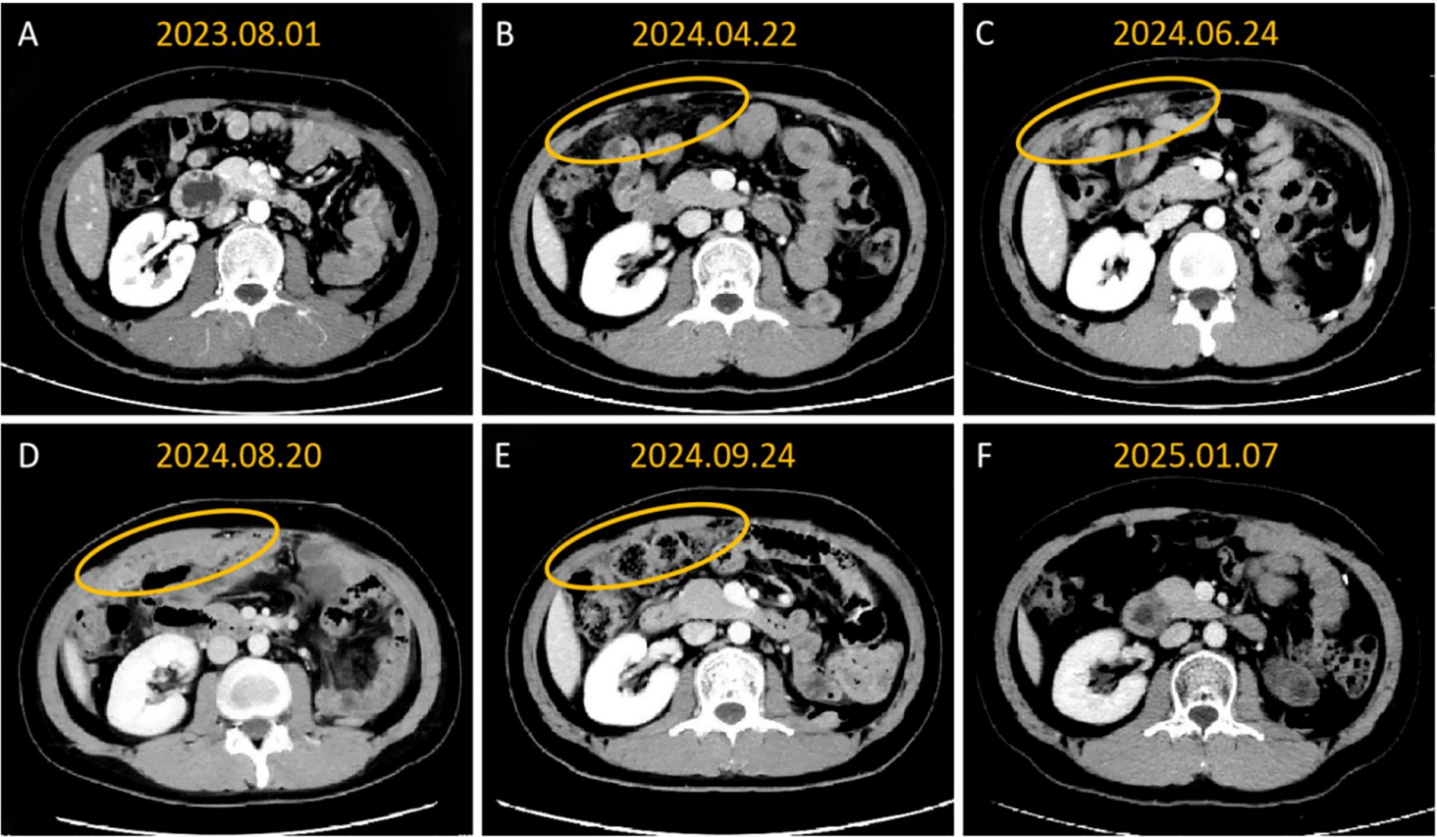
Contrast-enhanced CT imaging showed that sequential targeted therapy with intraperitoneal artery infusion of bevacizumab resulted in sustained partial remission of greater omental metastasies. (A) The abdominal cavity shows postoperative changes; (B) The greater omentum begins to show thickening and turbidity, suggesting recurrence and metastasis; (C) The metastatic tumor in the greater omentum has enlarged; (D) The lesion in the greater omentum is significantly thickened in a pancake-like manner; (E) The lesion in the greater omentum has significantly shrunk; (F) The greater omentum lesion has receded and is not clearly visible.
